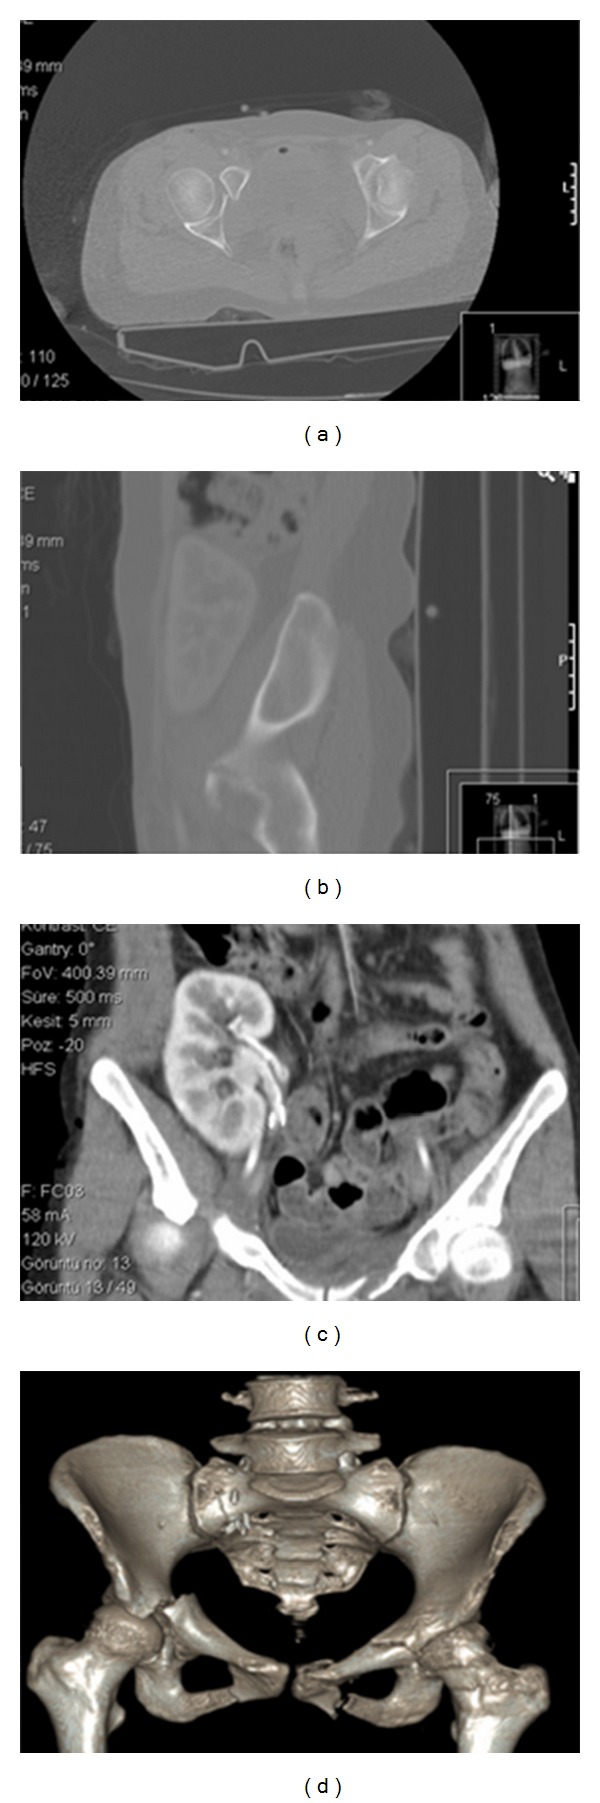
(a, b) Computed tomography (CT) showing the fracture of acetabulum. (c) Transplanted kidney is seen at coronal section of CT. (d) 3D CT view of fracture.
